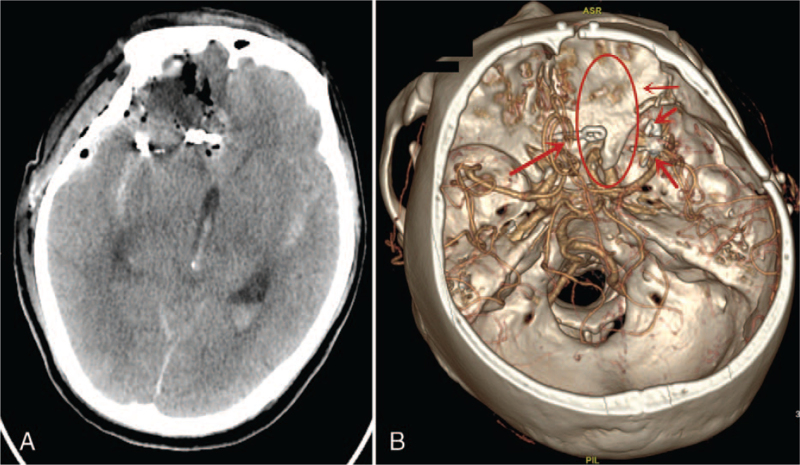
A and B: Are postoperative CT scan and CTA. A: Postoperative CT scan showing no intracranial hemorrhage. B: Postoperative CTA showing no AVM and a clip of the ACA in situ. Red circle and red thin arrow = location of AVM, red arrows = location of IAs and clip.
